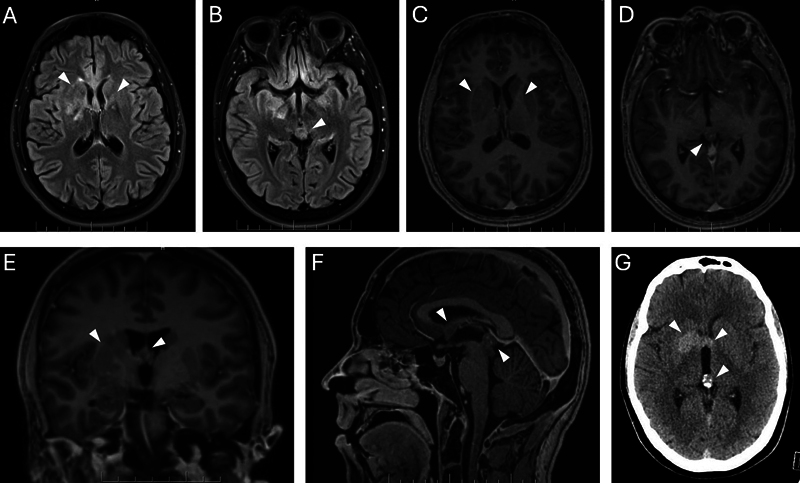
Magnetic resonance imaging (MRI) of intracranial germinomas in midline suprasellar and pineal regions, bilateral basal ganglia, fornices and hippocampi. Axial T2-fluid-attenuated inversion recovery-weighted MRI demonstrating areas of ill-defined T2-hyperintense lesions involving bilateral (R > L) basal ganglia, fornices, and pineal gland (
